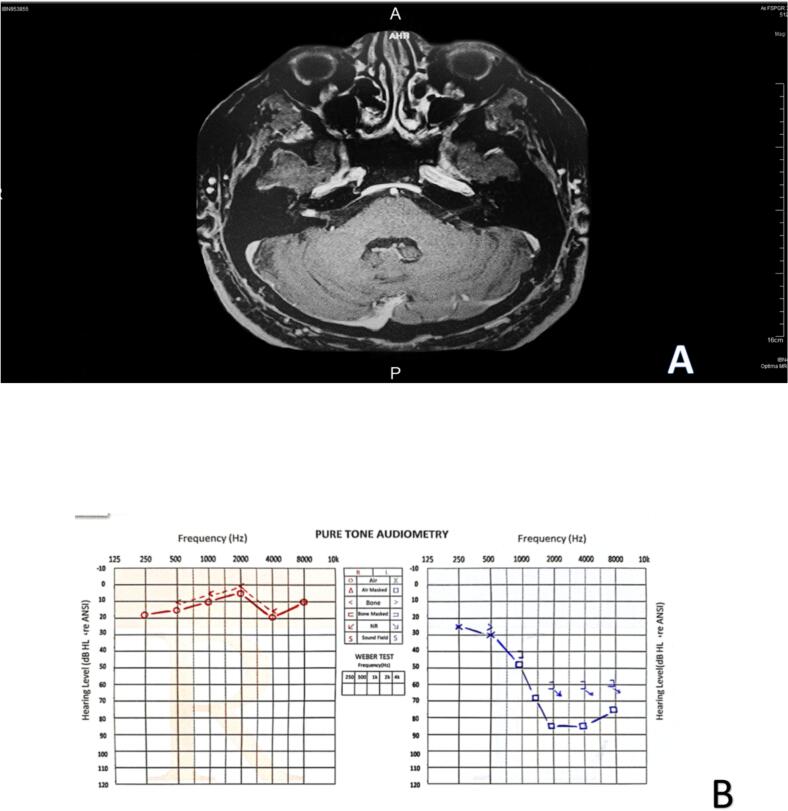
Case 2. A: The MRI revealed a small enhancing mass lesion measuring 5 mm, seen within the distal part of the right internal auditory canal. B: Pure tone audiometry demonstrated severe sensorineural hearing loss in the left ear at frequencies above 500 Hz and normal thresholds in the right ear.
